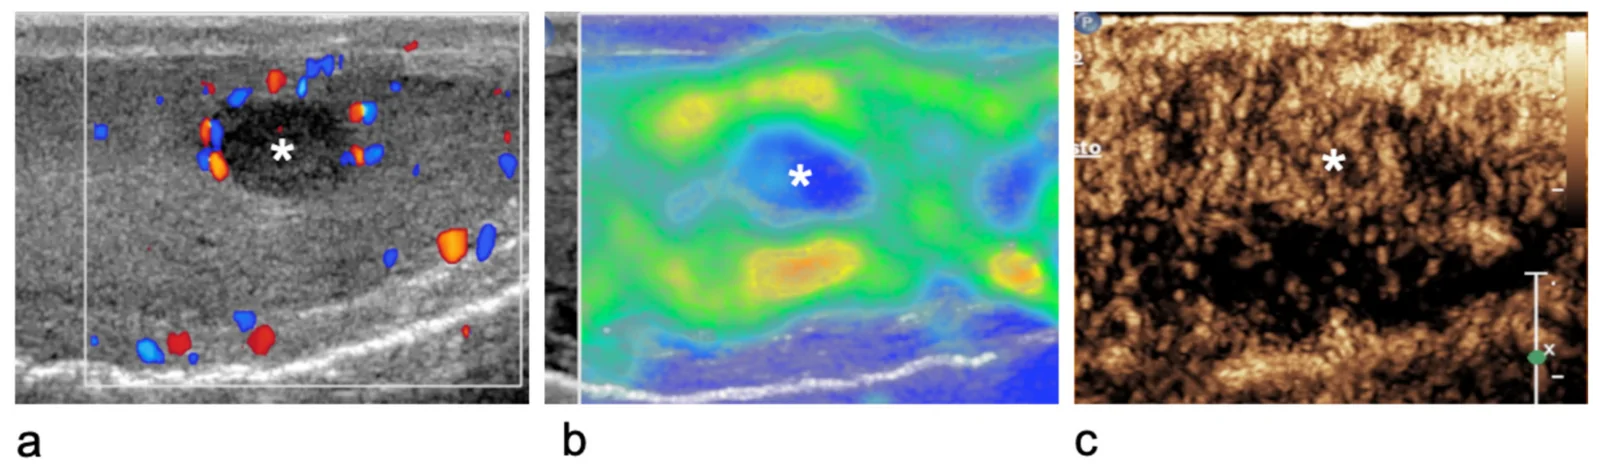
A 40-year-old man underwent ultrasonography after self-palpation of a firm lesion in the right testis. (a) Grey-scale and colour Doppler ultrasound show a small hypoechoic lesion (asterisk) with no apparent vascularity on colour Doppler imaging. (b) On elastography, the lesion (asterisk) appears stiff, in keeping with the palpable finding. (c) CEUS demonstrates hypervascularity within the lesion (asterisk). At surgery, the lesion proved to be a pure seminoma.
